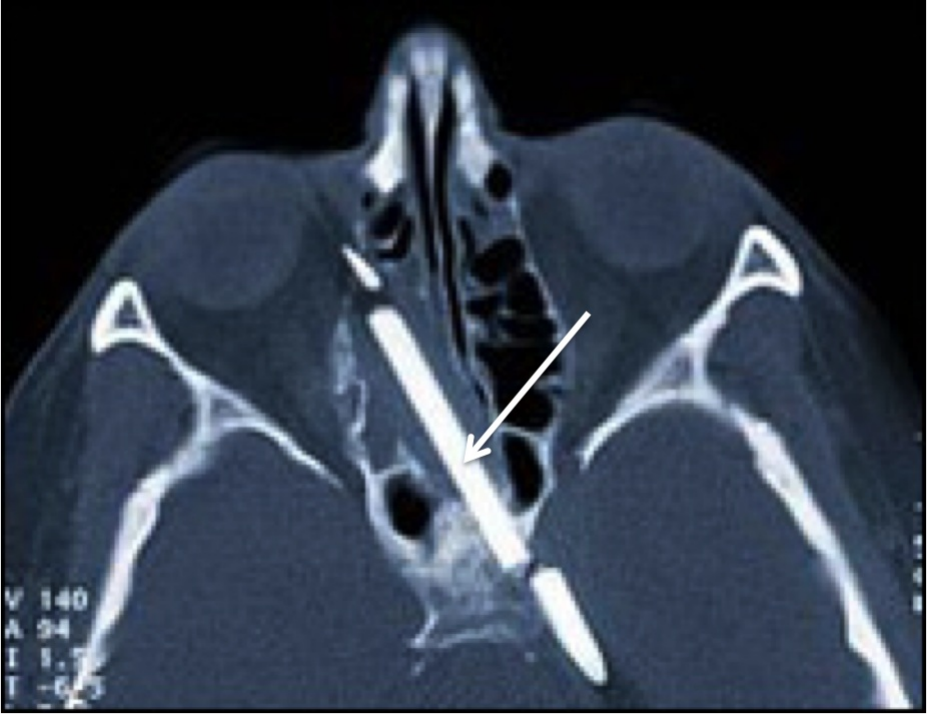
Axial head CT of a female child who fell onto a metal rod that entered the right medial orbit, traversed the ethmoid sinuses, and entered the left middle cranial fossa just lateral to the sella turcica. CT: computed tomography
